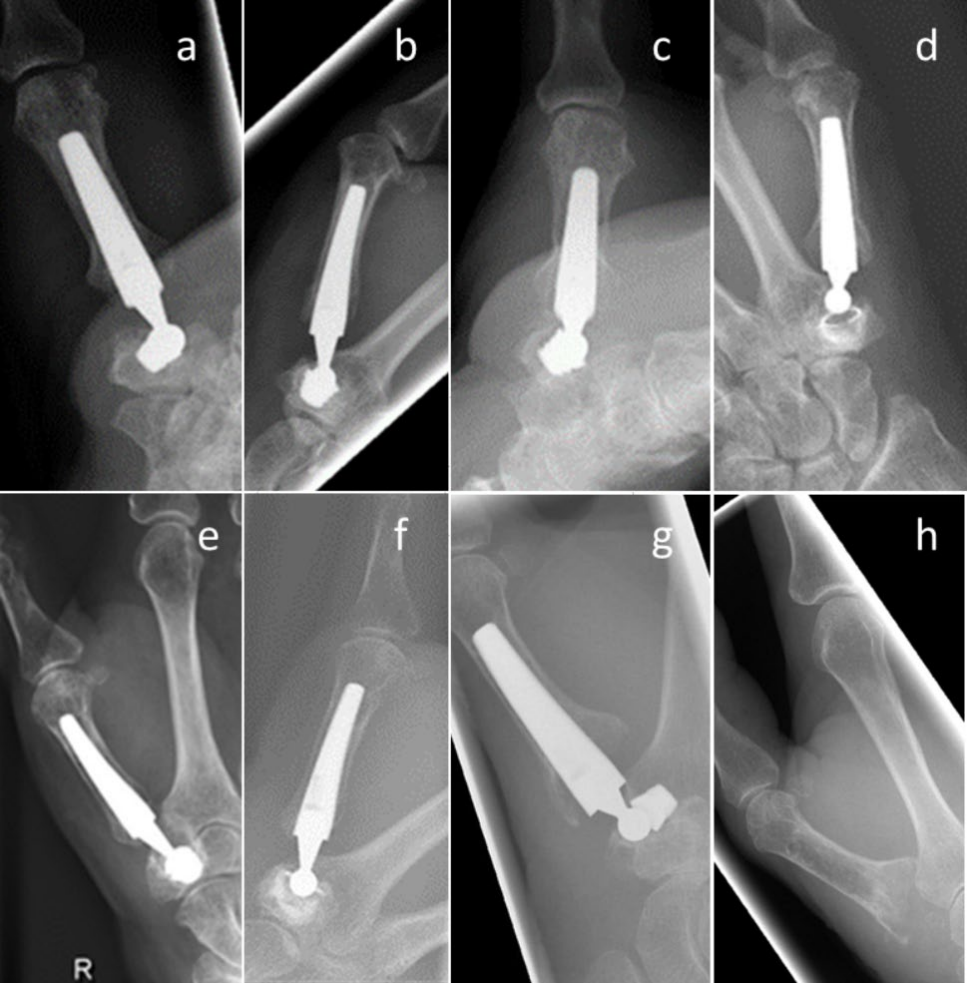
Radiographic imaging of adverse events in 3 patients with an Elektra prosthesis before and after revision surgery. Patient 1: (a) Cup loosening, treated with replacement of the cup and neck component (b) 6 months after primary surgery. (c) Second cup loosening in the same patient treated with replacement of the cup and neck component (d) 51 months after primary surgery. Patient 2: (e) Cup loosening treated with replacement of the cup and neck component (f) 84 months after primary surgery. Patient 3: (g) Cup loosening treated with secondary trapeziectomy (h) 18 months after primary surgery
